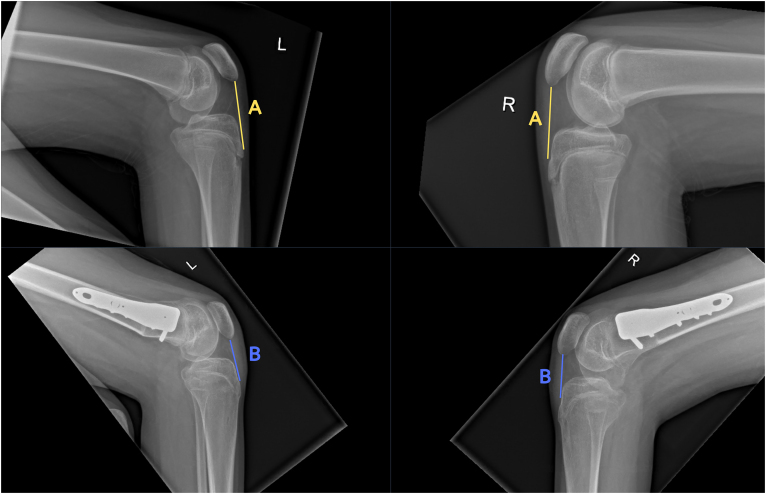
X-rays showing patella tendon plication achieved and maintained four months following bilateral distal femoral extension osteotomies. Preoperative patella tendon length (A) is greater than postoperative patella tendon length (B).
